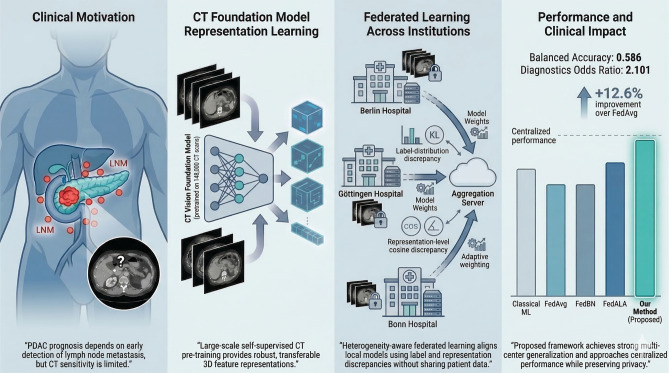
Overview of the proposed framework. A CT foundation model provides robust 3D representations for (LNM) detection in PDAC. Heterogeneity-aware FL enables collaborative training across institutions while preserving patient privacy and mitigating cross-site domain shift.
